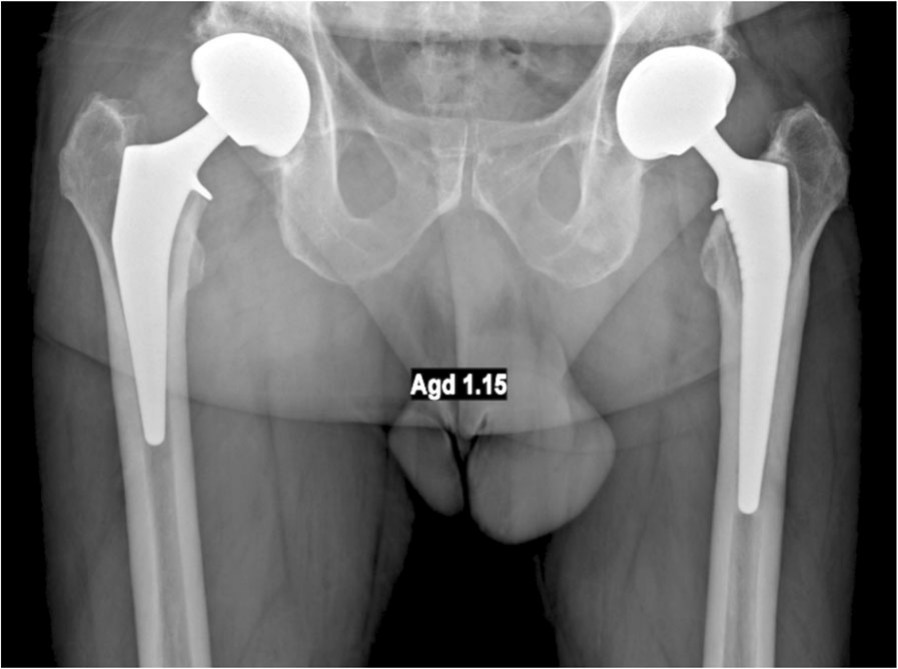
Postoperative anteroposterior radiograph of both implants, implanted in the same patient. The Symbol (shortened stem) is on the left and the Hype (standard stem) on the right
